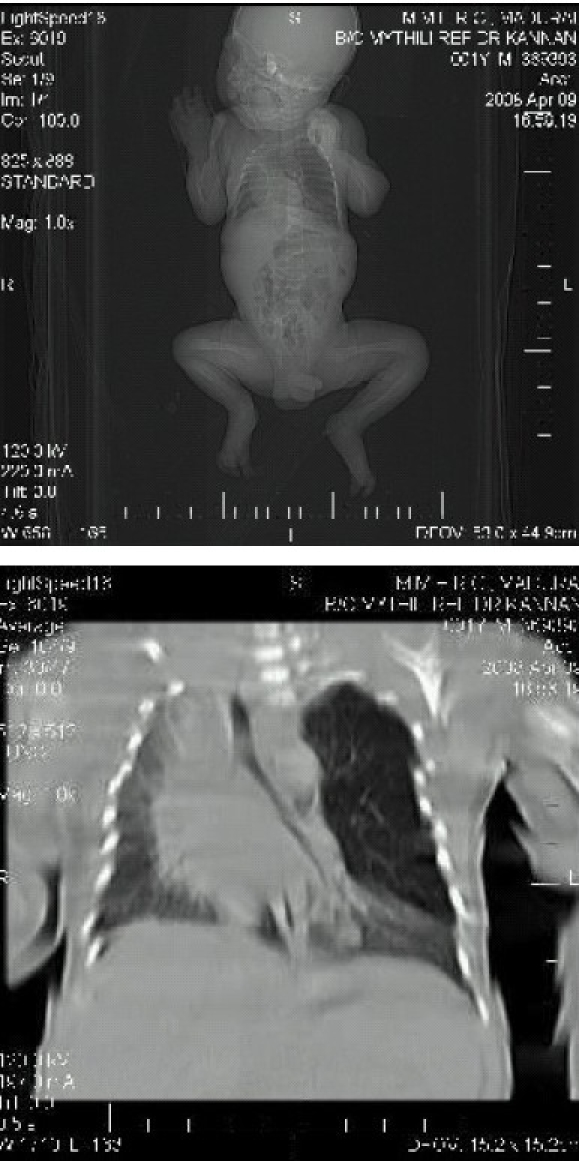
CT thorax showing hyperlucent, hyperexpanded left upper lobe causing compression of the remaining lung and mediastinal shift to right.
